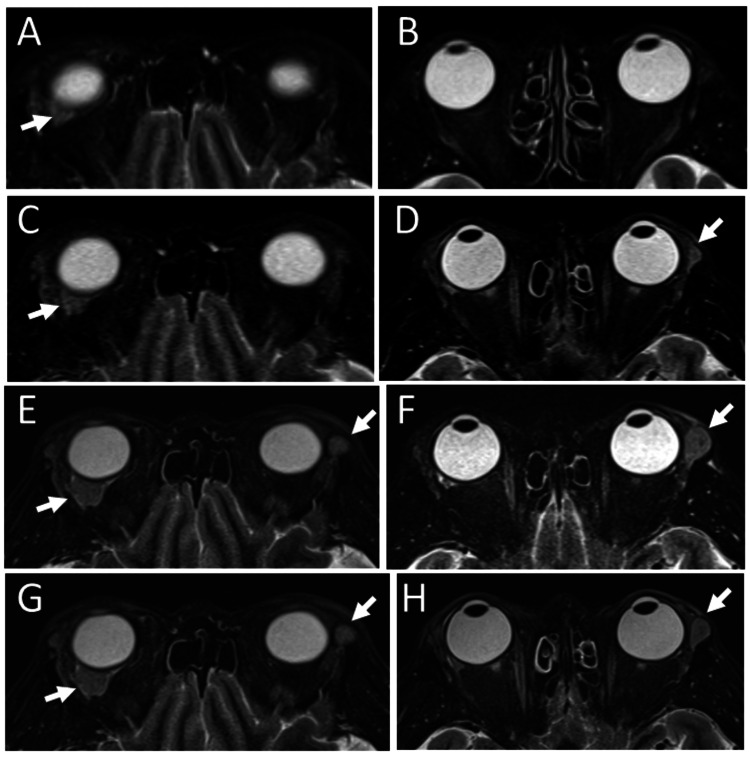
Magnetic resonance imaging at age 51 to 53 T2-weighted STIR (short tau inversion recovery) magnetic resonance imaging (A, B) 2 months later from excisional biopsy of right orbital mass at age 51, showing only a small residual mass (arrow, A) in the right upper orbit. Stable residual lesion on the right upper orbit (arrow, C) and a new mass lesion in the left superior lateral orbit (arrow, D), at age 52, 8 months later from the right excisional biopsy. The enlargement of the right superior orbital mass (arrow, E) and the left lateral orbital mass (arrow, F) 14 months from the right excisional biopsy. At age 53, just before left orbital mass excision, 20 months from the right orbital excisional biopsy, the same mass lesions are detected in bilateral orbits (arrows, G, H).
